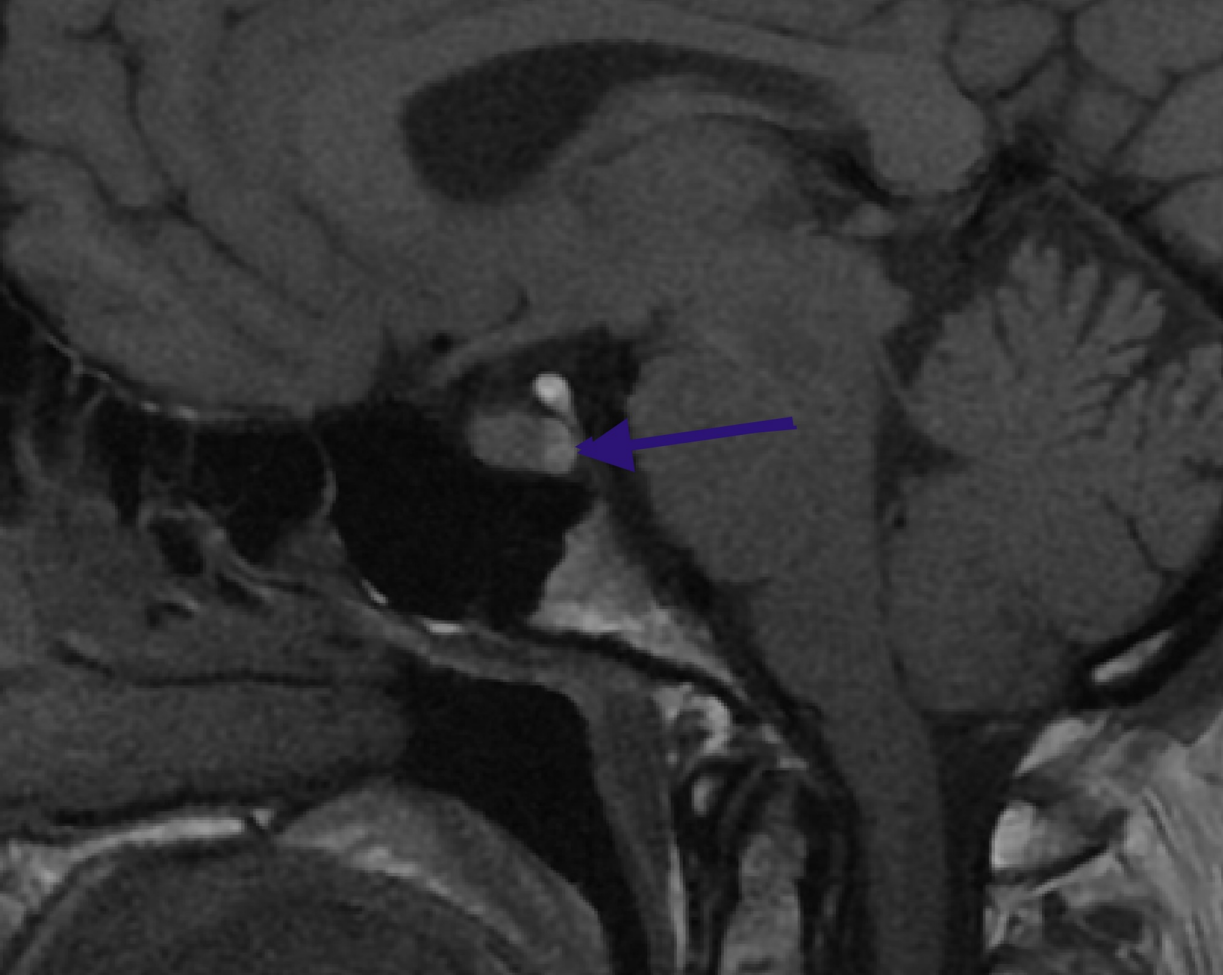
T1-weighted sagittal MRI: normal posterior pituitary hyperintense signal (blue arrow) MRI: Magnetic Resonance Imaging
